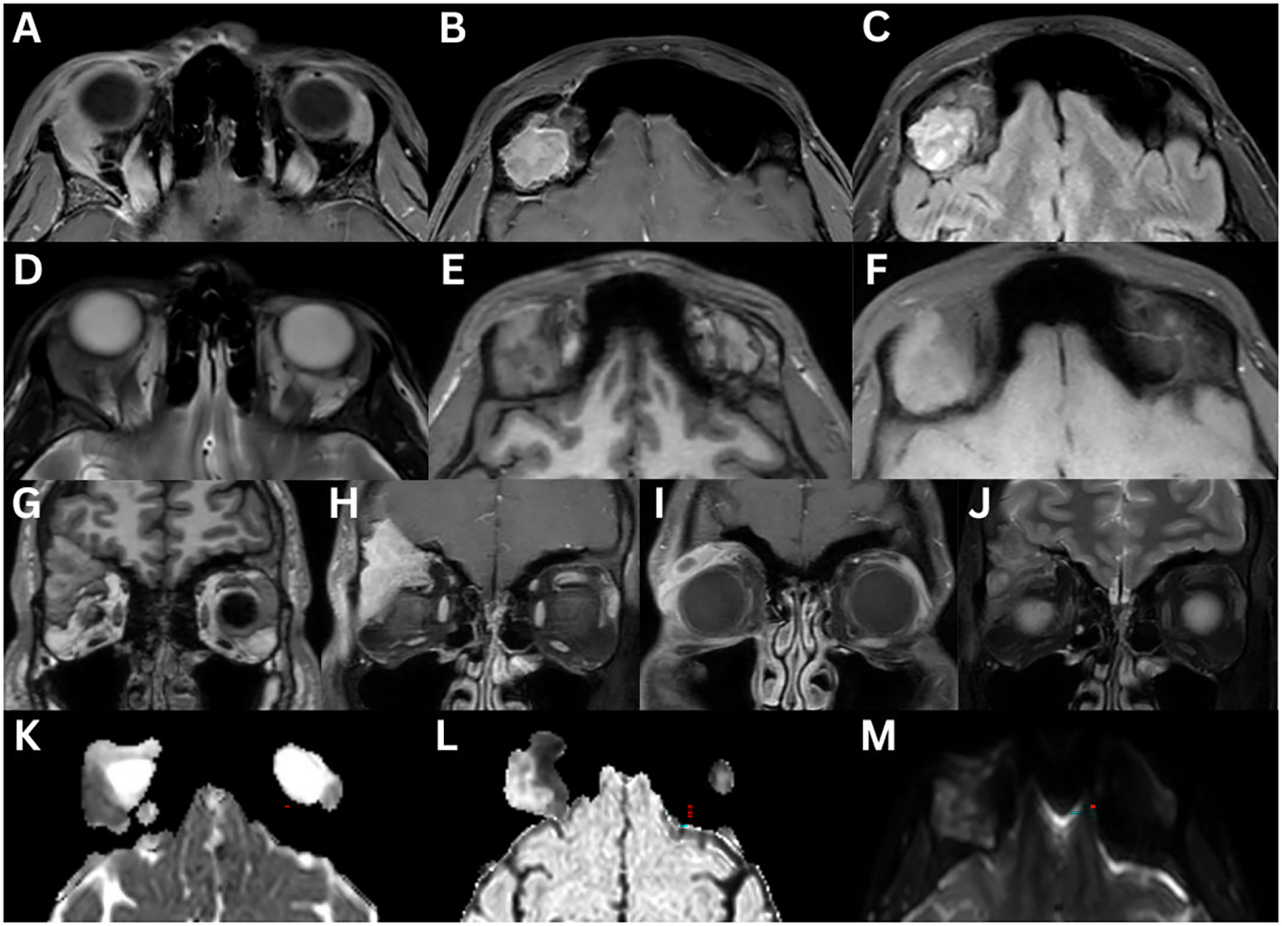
Langerhans cell histiocytosis with involvement of the right lacrimal gland. MRI axial T1-weighted fat-suppressed and contrast-enhanced (A, B), axial contrast-enhanced FLAIR SPIR (C), T2-weighted axial (D), T1-weighted axial (E), axial fat-suppressed (F), coronal (G) and coronal contrast-enhanced DIXON (H, I), and T2-weighted coronal DIXON (J) sequences demonstrate a heterogeneous mass with heterogeneous enhancement following contrast administration. The mass is isointense on T1-weighted imaging and mildly hyperintense on T2-weighted imaging, relative to adjacent extraocular muscles. There is restricted diffusion on axial ADC (K), axial eADC (L) and axial DWI SENSE (M).
